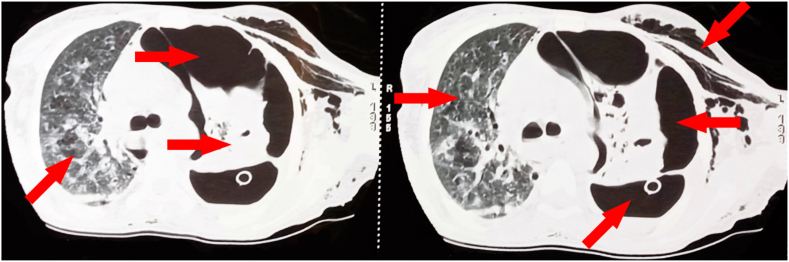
Chest CT scan showing a sequellae lung with left pneumothorax.
